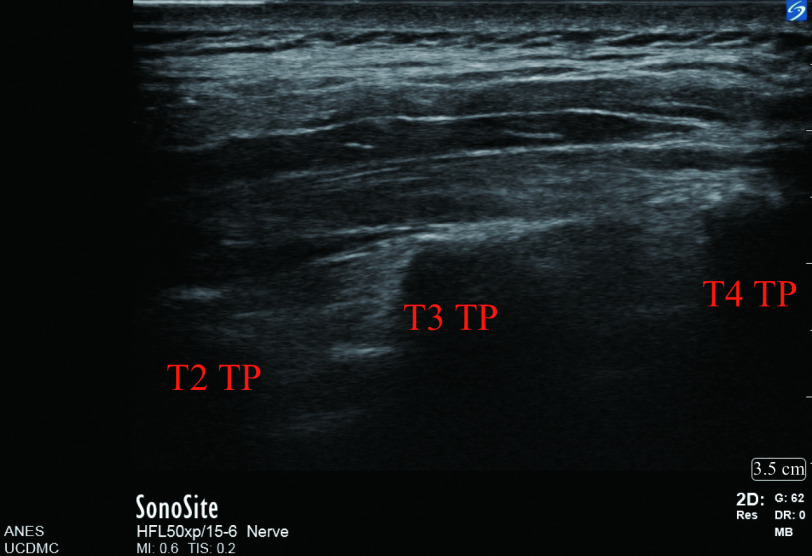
Ultrasound image of the paravertebral block.
